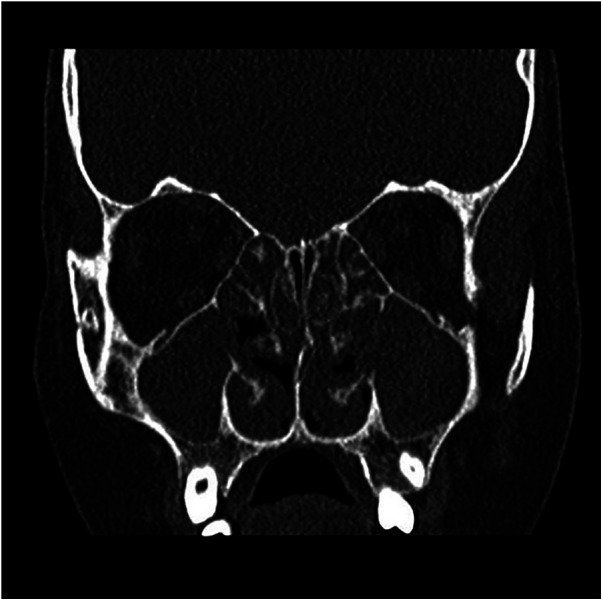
Coronal CT scan: almost complete opacification of the nasal cavities, the ethmoid labyrinth, and the maxillary sinuses.
